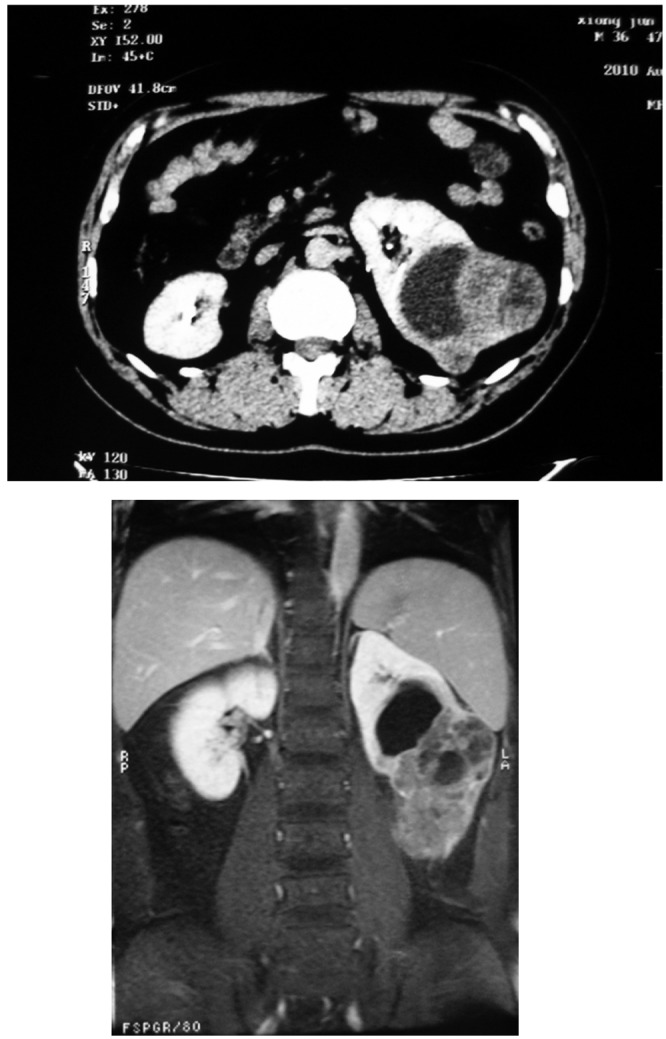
Computed tomography and magnetic resonance imaging showing a large solid mass located in the middle and lower pole of the left kidney with hydronephrectasis.
